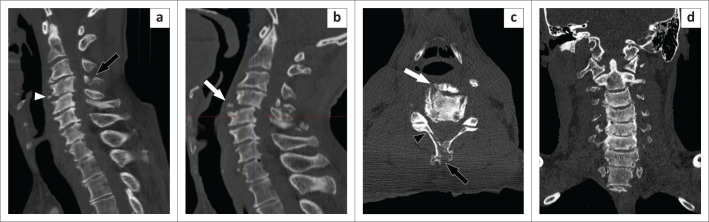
Sagittal reconstruction of the initial CT of the cervical spine (a) showing the C4 spinous process fracture (black arrow). The follow-up CT (b) shows worsened retrolisthesis of the C4 on C5 vertebra with an anterior teardrop fracture of the C4 vertebra (white arrow). This fracture is visible in (a) but was called a ‘fractured osteophyte’ (white arrowhead). The axial (c) and coronal (d) reconstructions of the scan show how the degenerative cervical spine changes may make diagnosing fractures challenging – the teardrop fracture is pointed out by the white arrow in (c) and may have been misinterpreted as an osteophyte (white arrowhead). The black arrowhead in (c) demonstrates a right lamina fracture, and the black arrow again points out the spinous process fracture.
